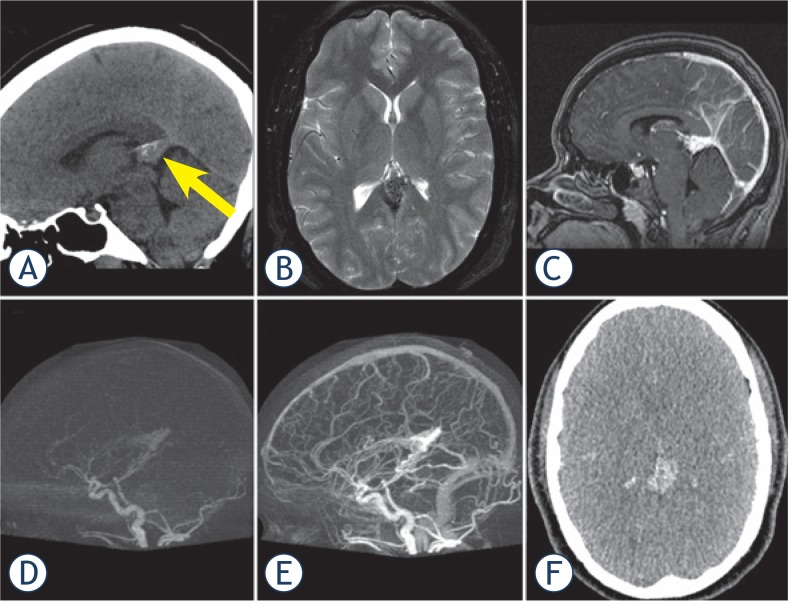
(A) Sagittal reformatted non-contrast CT head image showing iso-dense lesion in tentorial notch (arrow). (B) Axial T2 weighted MR image (3B) showing nidus with adjacent draining vein. (C) Sagittal reformatted image from T1 weighted VIBE image showing enhancing nidus with adjacent draining veins and venous sinuses. (D–E) Sagittal reformatted subtracted dCTA images in arterial (D) and venous (E) phase showing the AVM nidus in the arterial phase and occluded straight sinus. (F) Axial image from early arterial volume showing the nidus without contamination from surrounding enhancing vessels (full CT dataset from this temporal imaging phase imported into CBK for contouring).
